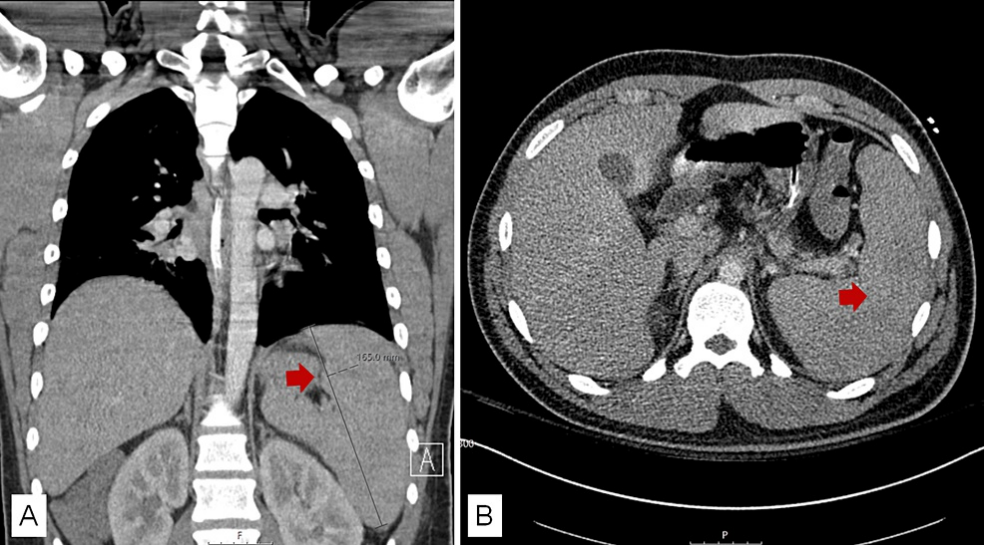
Abdomen CT scan coronal (A) and axial (B) views Splenomegaly (red arrow).
